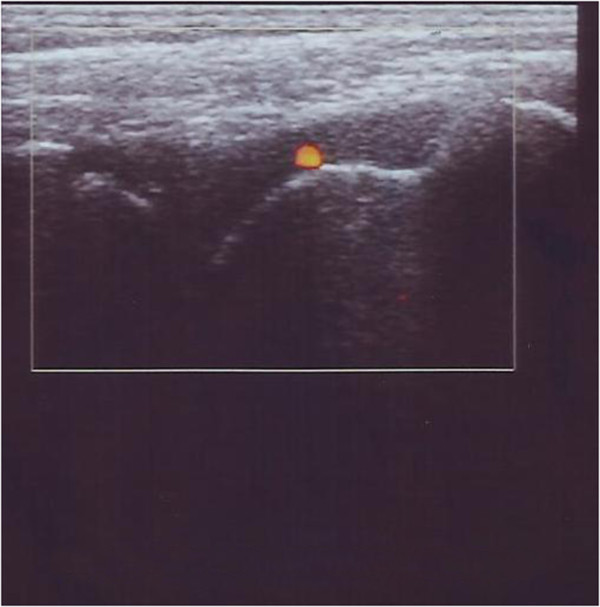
Case 1 left ankle ultrasound. Midsagittal 12–5 MHz ultrasound image over the dorsal ankle shows a distended anterior joint recess, filled with hypoechoic fluid with active synovial pannus as marked by color Doppler examination.
